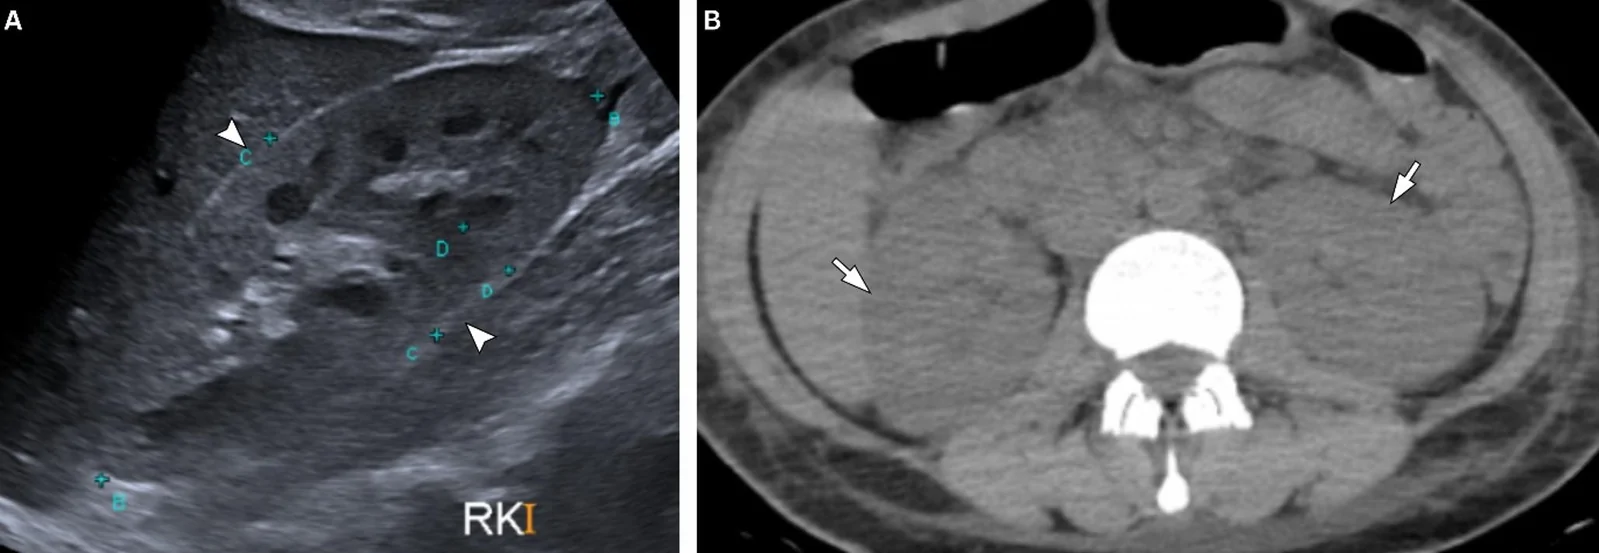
Renal involvement in scrub typhus in a 29-year-old male with rising creatinine and bilateral patchy consolidation. A Longitudinal transabdominal ultrasound of the right kidney demonstrates renal enlargement with increased cortical echogenicity (white arrowheads). B Unenhanced axial CT of the abdomen shows bilateral hypoattenuating renal parenchyma (solid white arrows), consistent with acute kidney injury
